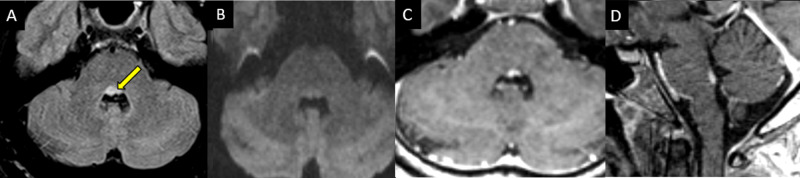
A 39-year-old male with a known clinical history of multiple sclerosis presented with bilateral INO, diplopia, and dizziness. (A) Axial FLAIR sequence demonstrates a lesion in the dorsal pons in the medial longitudinal fasciculus (arrow). (B) Axial DWI sequence demonstrates no restricted diffusion to suggest an acute infarct. (C, D) Axial and sagittal postcontrast T1 axial images demonstrate solid enhancement in this location. INO: internuclear opthalmoplegia, FLAIR: fluid-attenuated inversion recovery, DWI: diffusion-weighted imaging
